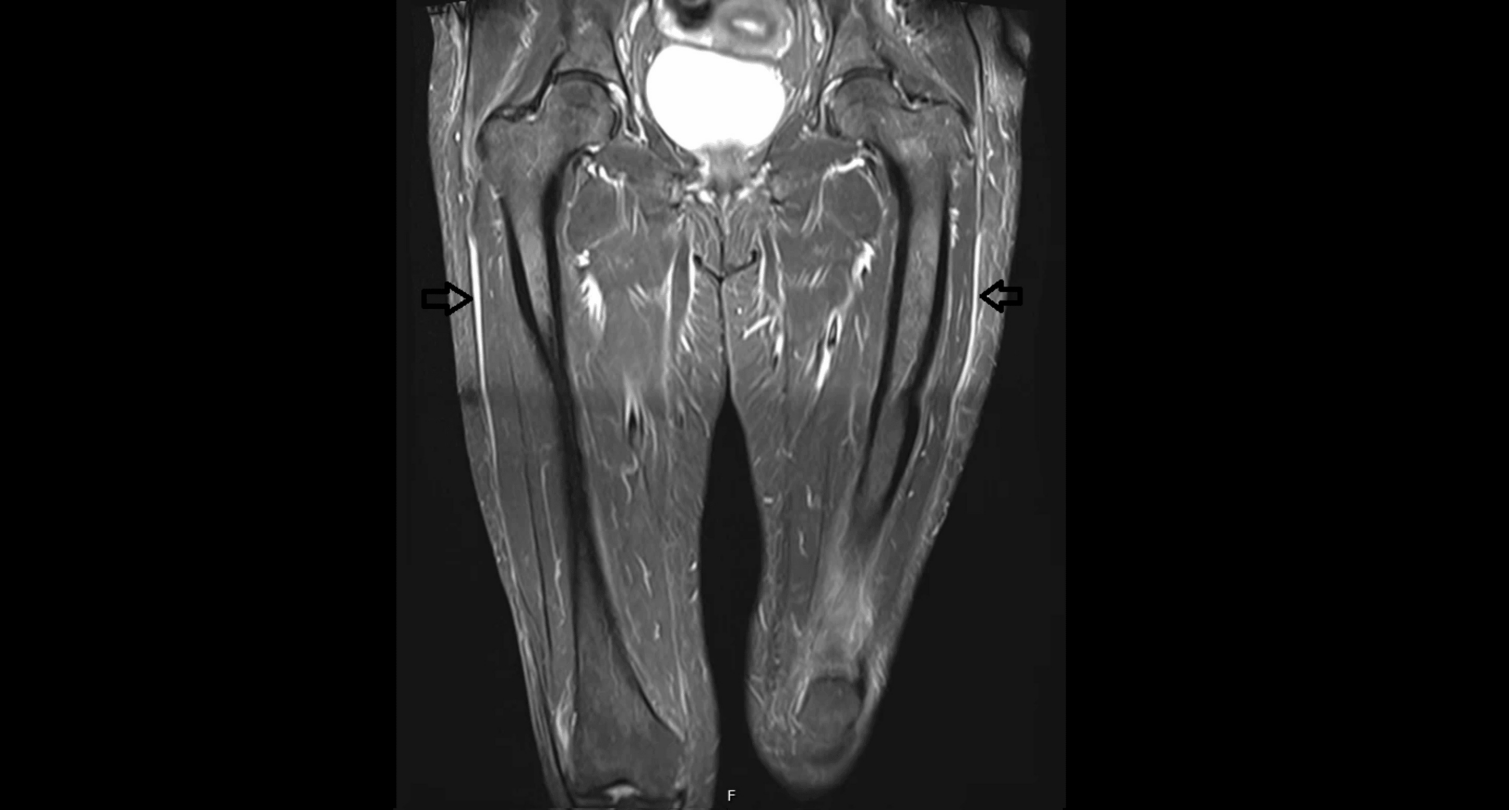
Coronal MRI scan of the hips using a STIR sequence, revealing fascial inflammation MRI: magnetic resonance imaging; STIR: short Tau inversion recovery
